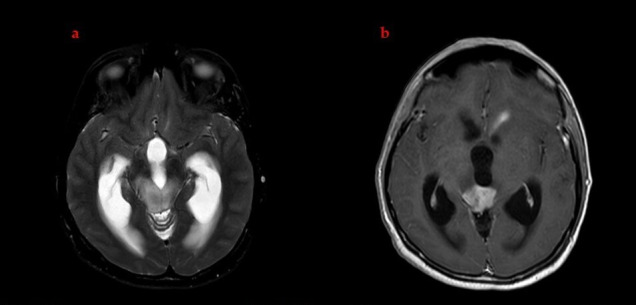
Pre-targeted therapy brain MRI demonstrating the baseline appearance of the lesion. (a) Axial T2-weighted image showing the tectal mass accompanied by ventriculomegaly. (b) Axial post-contrast T1-weighted image revealing heterogeneous enhancement of the lesion prior to initiation of mechanism-guided targeted therapy.
